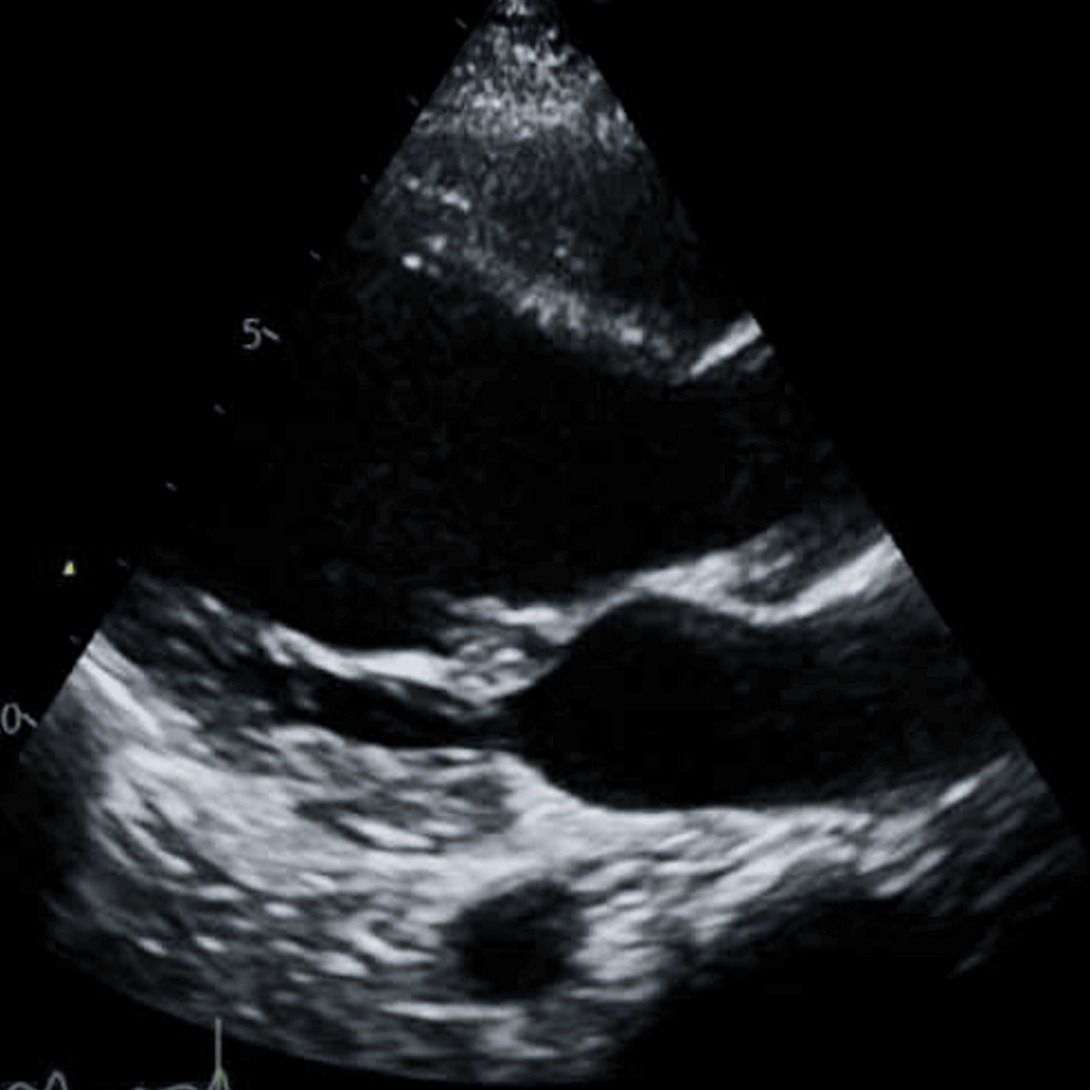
The bedside echo in its parasternal long axis view, which shows normal sized heart with fair contractility
